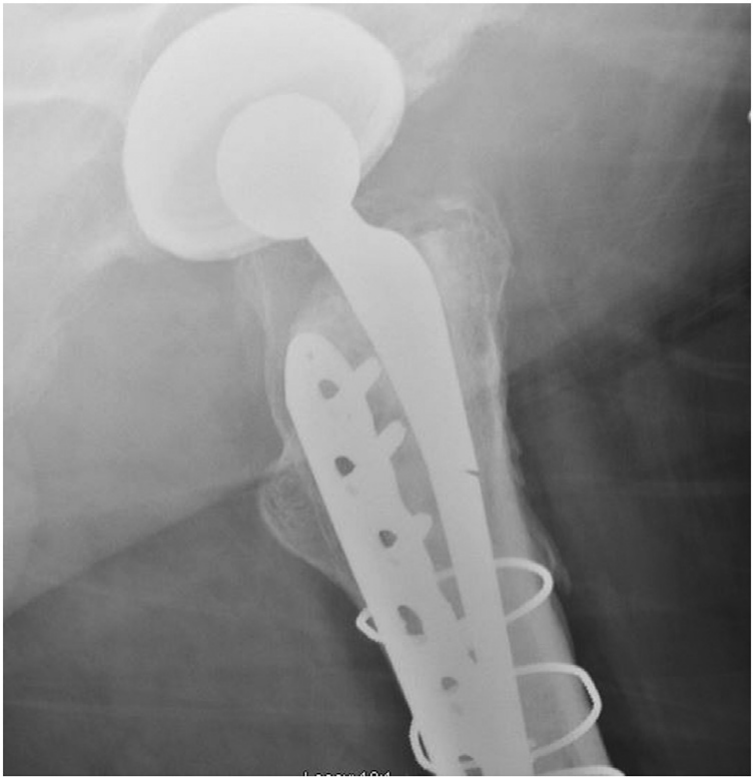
Lateral radiograph of the hip showing a stem fracture of a short Exeter stem.
